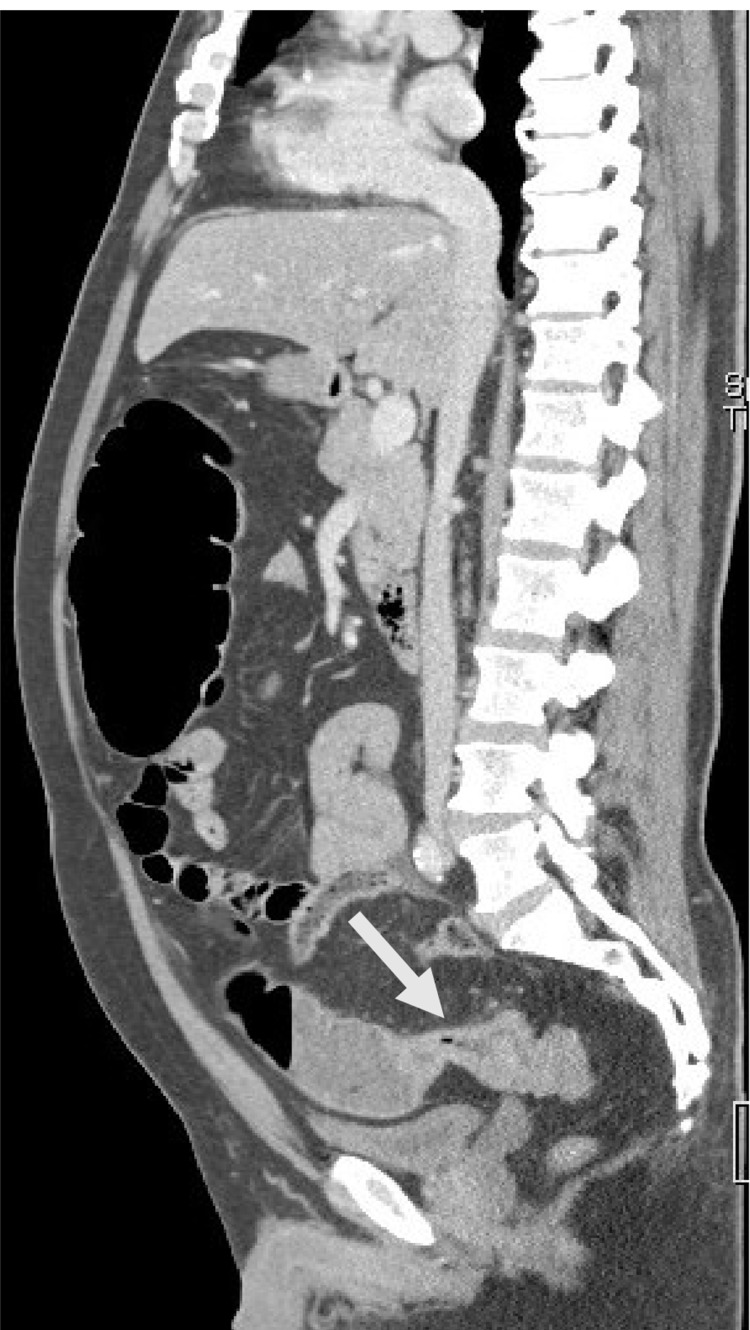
CT scan in the sagittal plane showing stricture (arrow) formed by the tumor at the rectosigmoid junction with proximal bowel dilatation.
